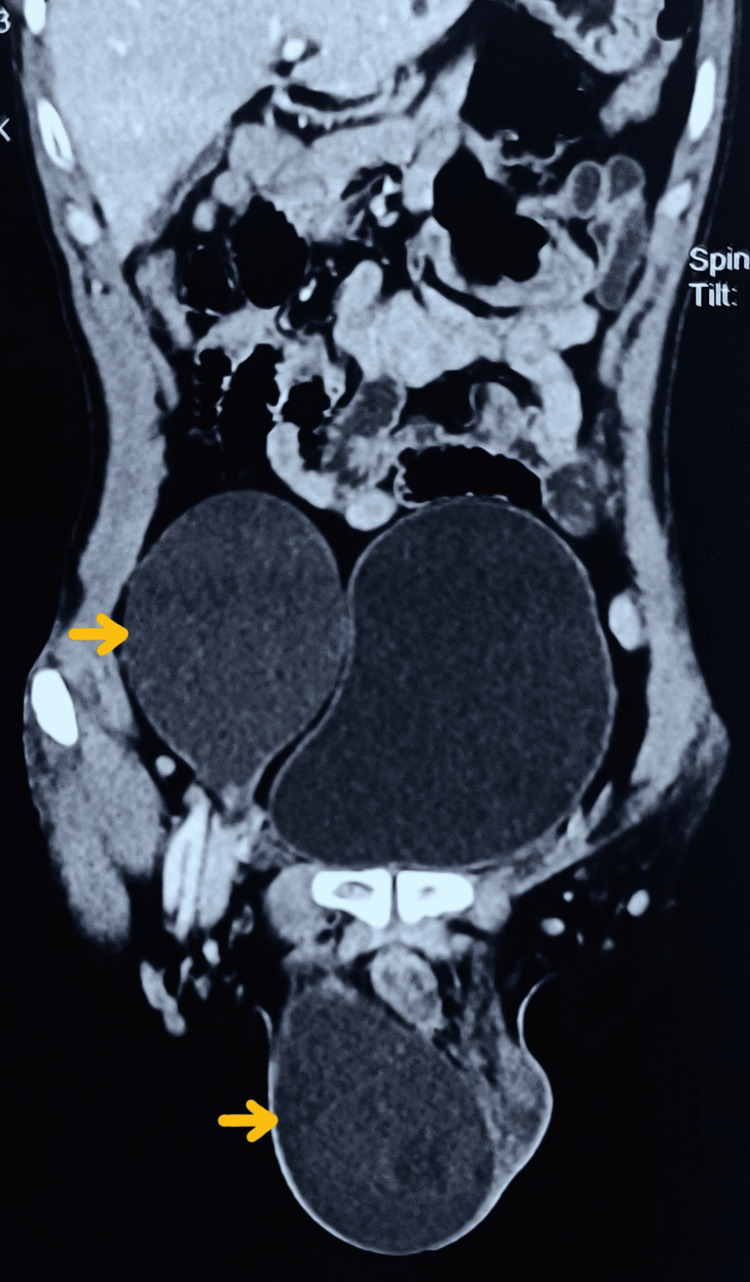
Contrast-enhanced computed tomography of the coronal section showing cystic abdominal swelling in continuity with the scrotal swelling (marked with yellow arrows).
